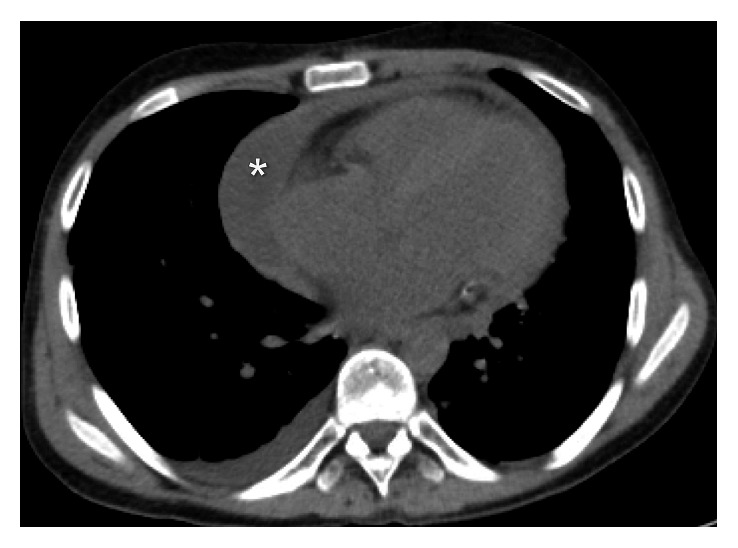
CT chest transverse view (pericardial effusion denoted by ∗).
